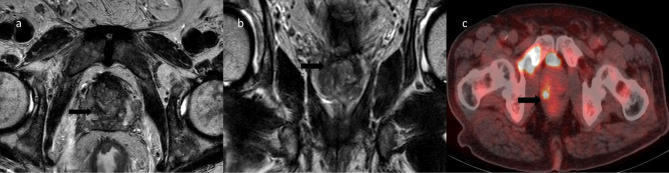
A significant increase in the metabolic activity of the incidental right peripheral prostate focus was detected in a 62-year-old patient who underwent 18F-FDG PET/CT for the staging of metastatic lung cancer and whose serum PSA was 2.36 ng/mL. In prostate mp-MRI, a lesion with an SUVmax of 3.5 was shown in the sagittal T2A MRI (a) in the area corresponding to the central zone in the right midapex and in the 18F-FDG PET/CT scan (c) in the coronal T2A MRI. It is compatible with the central zone
